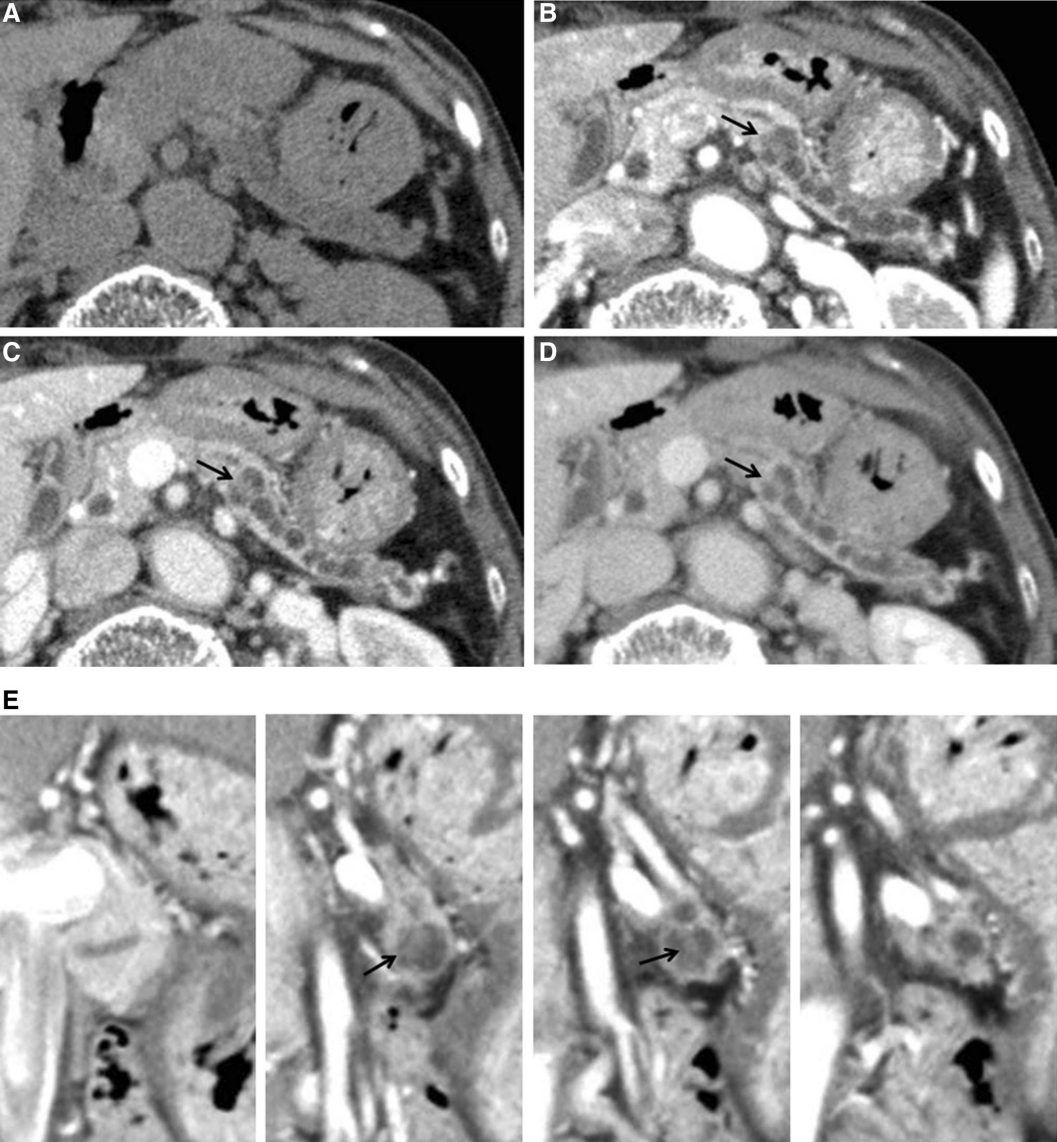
A–E Axial pre-enhanced (A) and axial multiphase dynamic contrast-enhanced (B pancreatic phase; C portal venous phase; D equilibrium phase) and coronal contrast-enhanced (E portal venous phase) CT images show a papillary mass at the downstream edge of the dilatated main pancreatic duct lumen (*arrows*). The mass is slightly enhanced between the pancreatic phase and delayed phase on a dynamic study. The duct is dilatated in the pancreatic body and tail, but not in the downstream of the mass. The surrounding pancreatic parenchyma in the body and tail is atrophic and its border with the mass is irregular.
